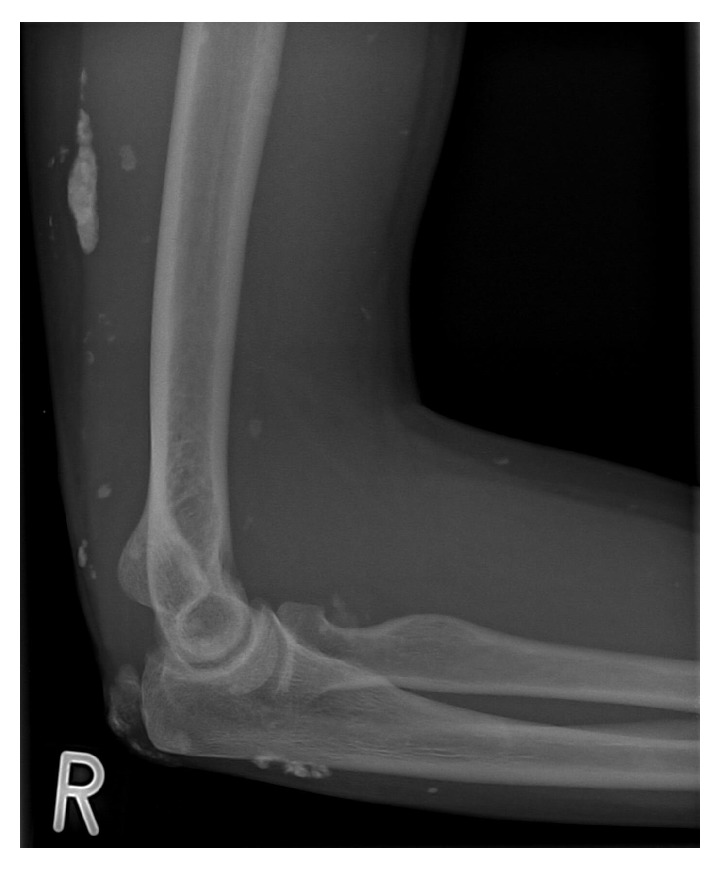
Standard lateral elbow radiograph of a 26-year-old woman 30 months after cautious broaching of large calcific lesions in calcinosis universalis associated with juvenile Dermatomyositis of the musculus triceps brachii and its tendon plus resection of an ulcerating sinus.
